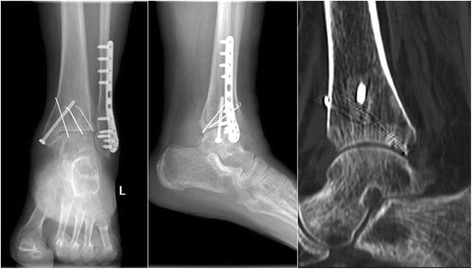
Postoperative X-ray and CT images 7 months after the surgery showed satisfactory fracture healing
